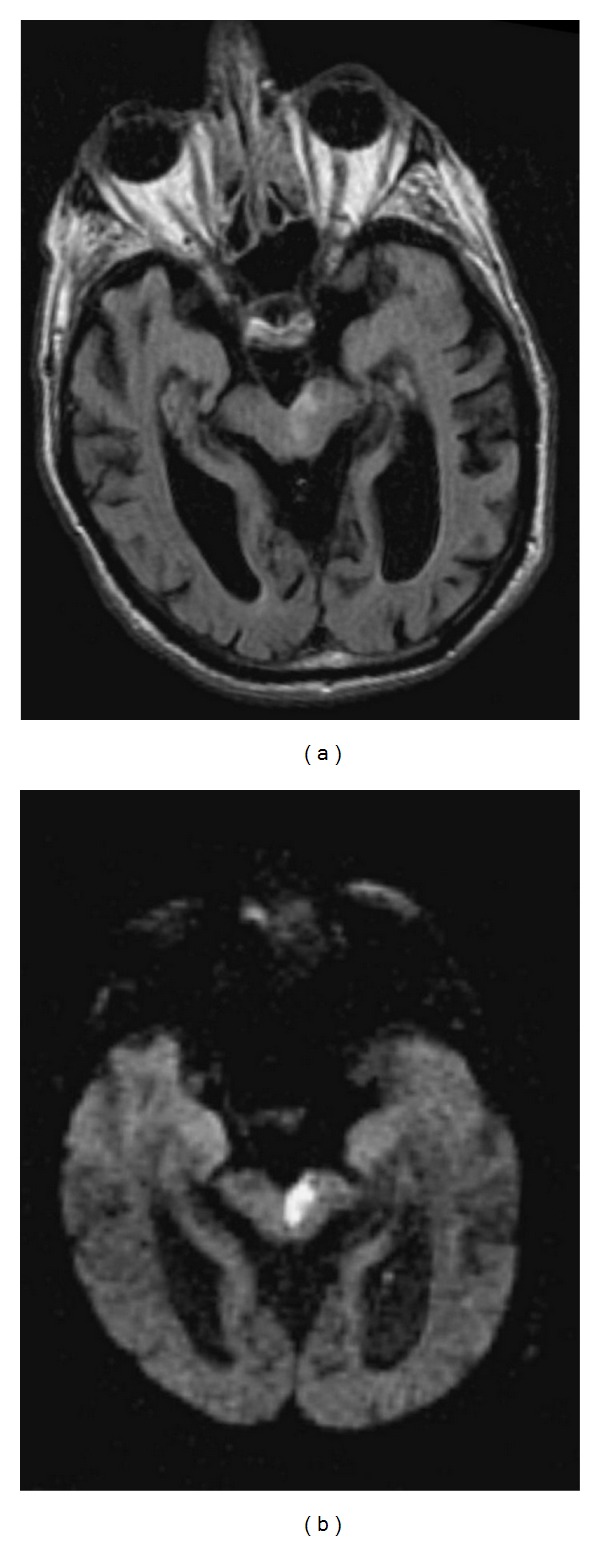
Weber's syndrome. FLAIR (a) and DWI (b) images showing a left paramedian midbrain infarct in a patient with Weber's syndrome. Images used courtesy of Dr. Frank Gaillard at Radiopaedia. org (http://radiopaedia.org/images/94/).
